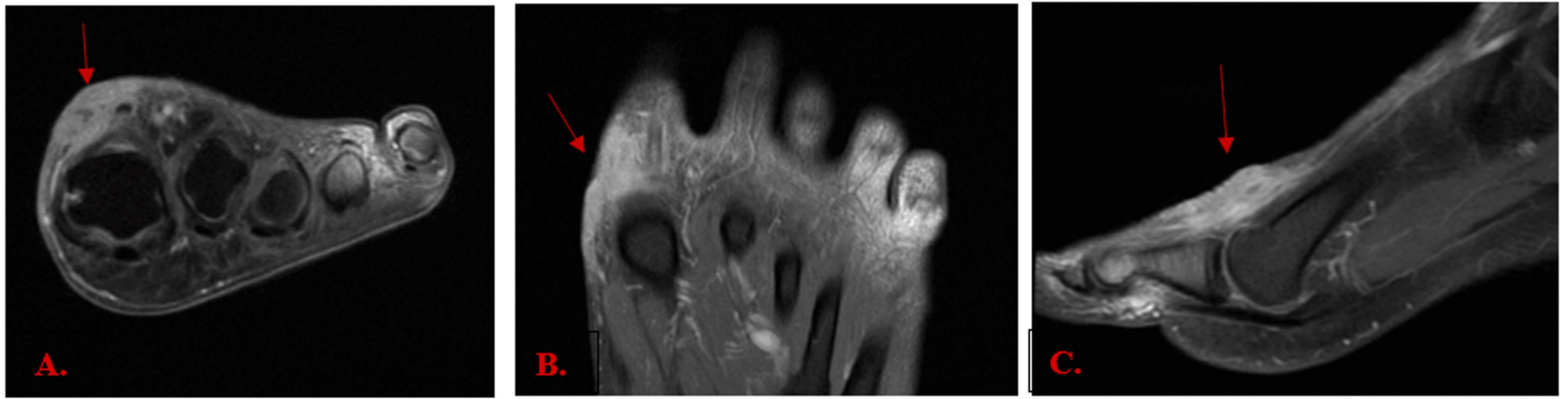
Post-contrast MRI images of the left foot Post-contrast left foot MRI images in the axial (A), coronal (B), and sagittal (C) planes showing diffuse enhancement of the dorsal skin and subcutaneous tissues (red arrows).
